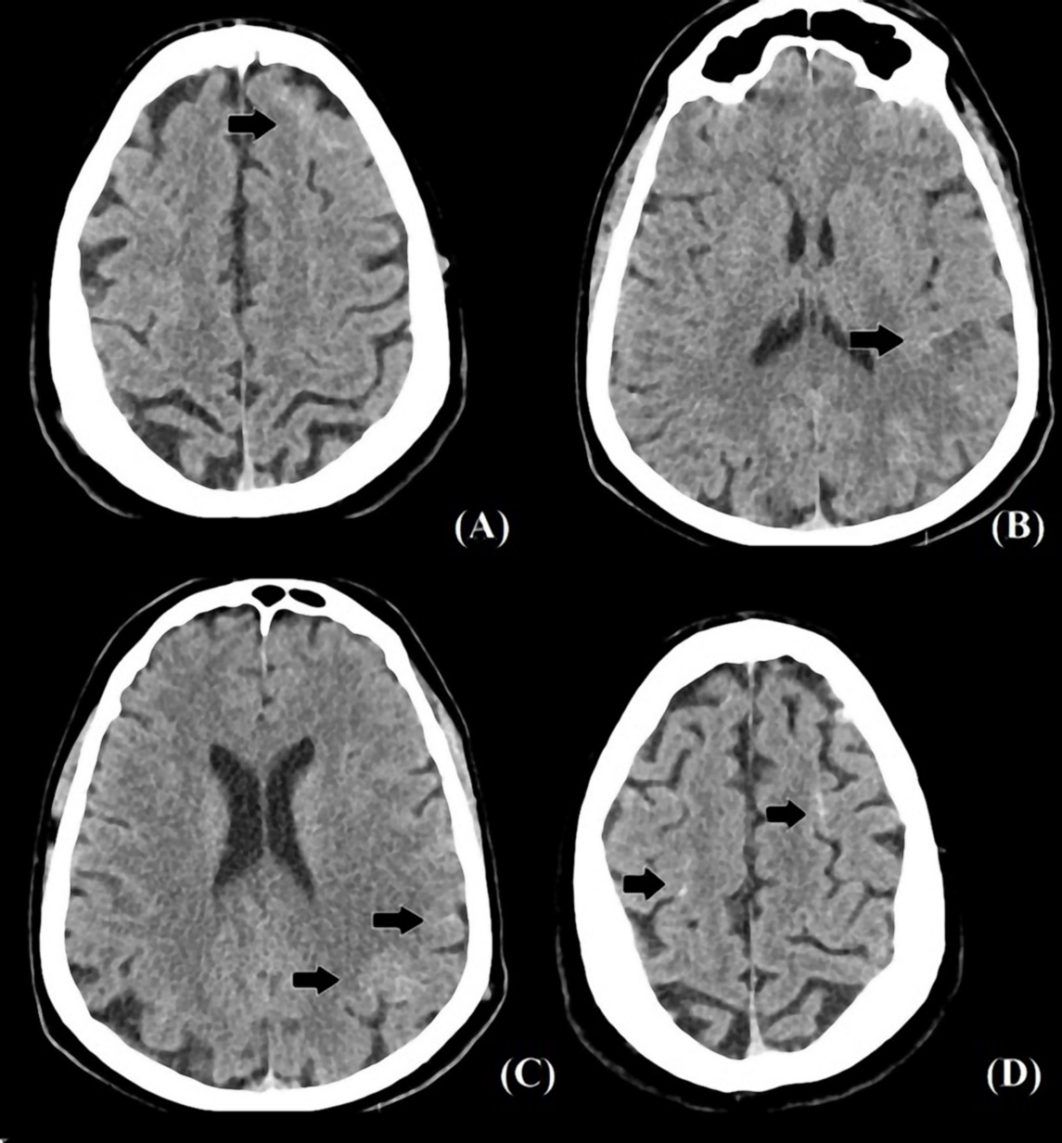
CT-scan, axial cross-sections of the brain. A, C, D - Arrows pointing to punctate subarachnoid hemorrhages. B - Arrow points to a small ischemic lesion with a narrow rim of hemorrhage.
